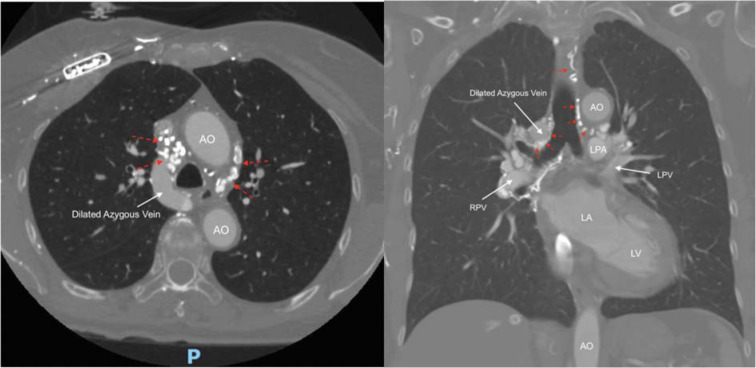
From left to right, axial and coronal segments of computed tomography angiography imaging of the heart; dashed red arrows indicate the network of collaterals directed toward bilateral pulmonary veins that had developed extensively across the chest. *Abbreviations:* AO, aorta; LA, left atrium; LPA, left pulmonary artery; LPV, Left pulmonary vein; LV, left ventricle; RPV, right pulmonary vein.
